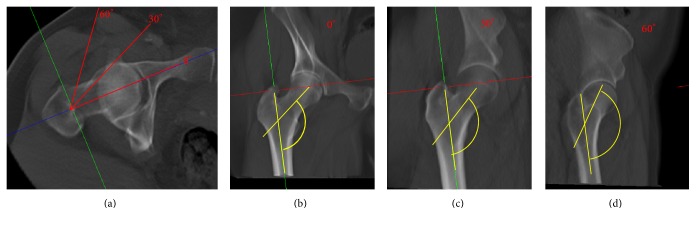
Effect of hip rotation on the projected neck-shaft angle (NSA). The axial view of the femur in a CT reconstruction of a right hip is shown (a). Three coronal reconstructions are projected. Internal hip rotation of 0°, 30°, and 60° is demonstrated as shown in the axial view. The NSA is included and the overestimation of the projected NSA due to the rotation is visualized.
